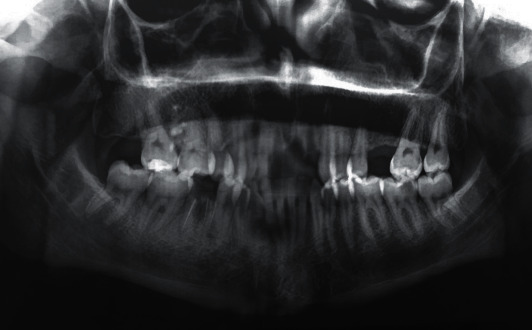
Preoperative panoramic radiograph showing empty sockets of 11, 21, and 22. No sign of fracture or contusion of the alveolar sockets.
